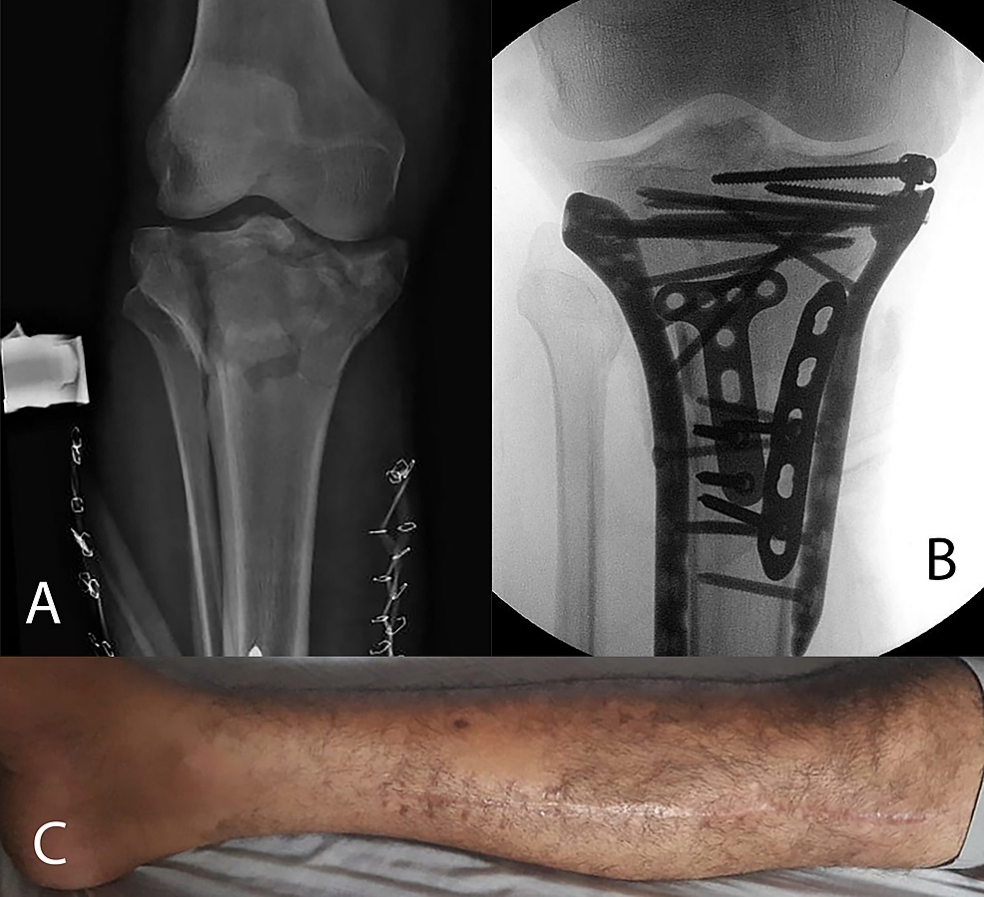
Definitive treatment. A: Post-operative anteroposterior radiograph showing the placement of a spanning external fixator to the right knee as well as the presence of rubber band lace-up technique on both sides of the leg. B. Intra-operative anteroposterior flouroscopic view showing definitive fixation of the right tibial plateau fracture. C. Clinical image showing healed scars and good soft tissue status.
